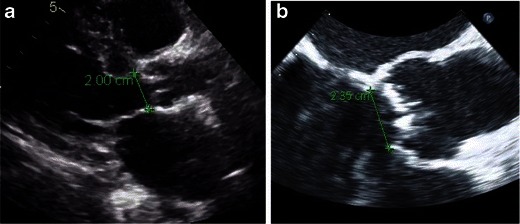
Aortic annular measurement by (a) transthoracic echocardiograpy: parasternal long-axis view with measurement shown. b Transesophageal echocardiography (TEE): long-axis view shown with annular measurement. Transthoracic echocardiography (TTE) typically underestimates the aortic annulus by approximately 1.0–1.5 mm when compared to TEE or MDCT
